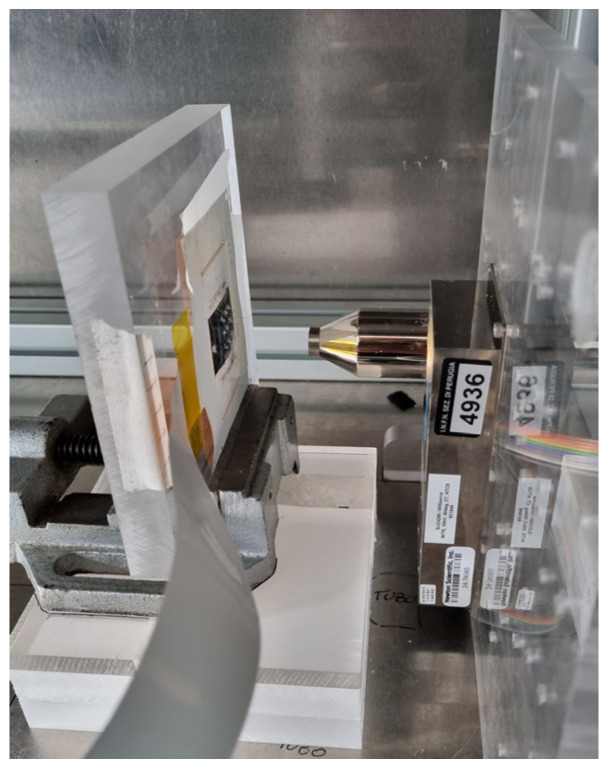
Setup for X-ray testing. The detector shown in Figure 2 is connected to the SMU through the PI PCB interface frame shown in the same figure. The picture also shows the X-ray tube. The entire setup is enclosed in a radiation-shielded cabinet.
